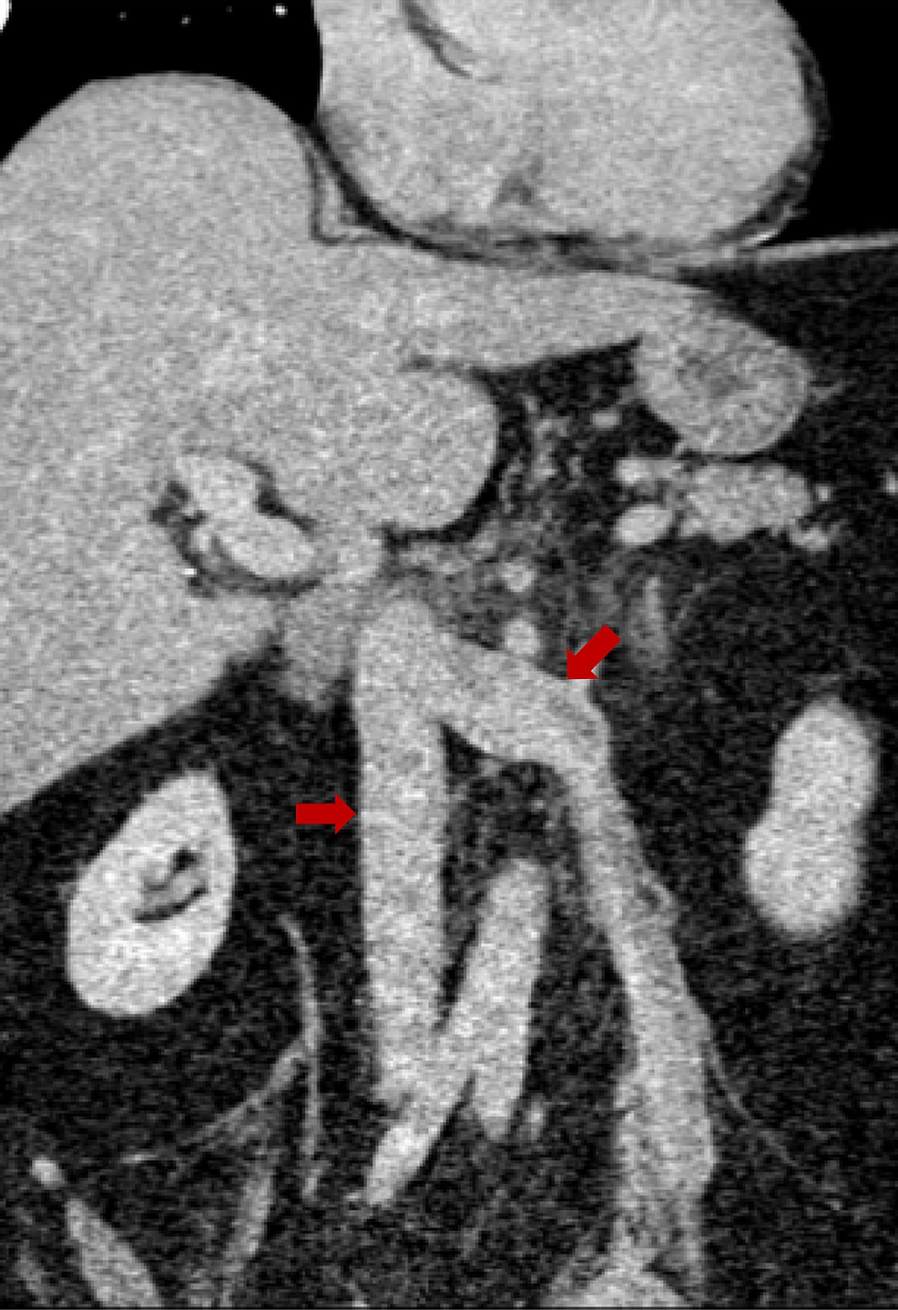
Coronal contrast-enhanced CT of the abdomen Continuous hypodense lesion extending from the left gonadal vein, through the left renal and into the IVC. Hypodensity in the right atrium, shown more clearly in the cardiac MR appears to be a continuation of the lesion.
